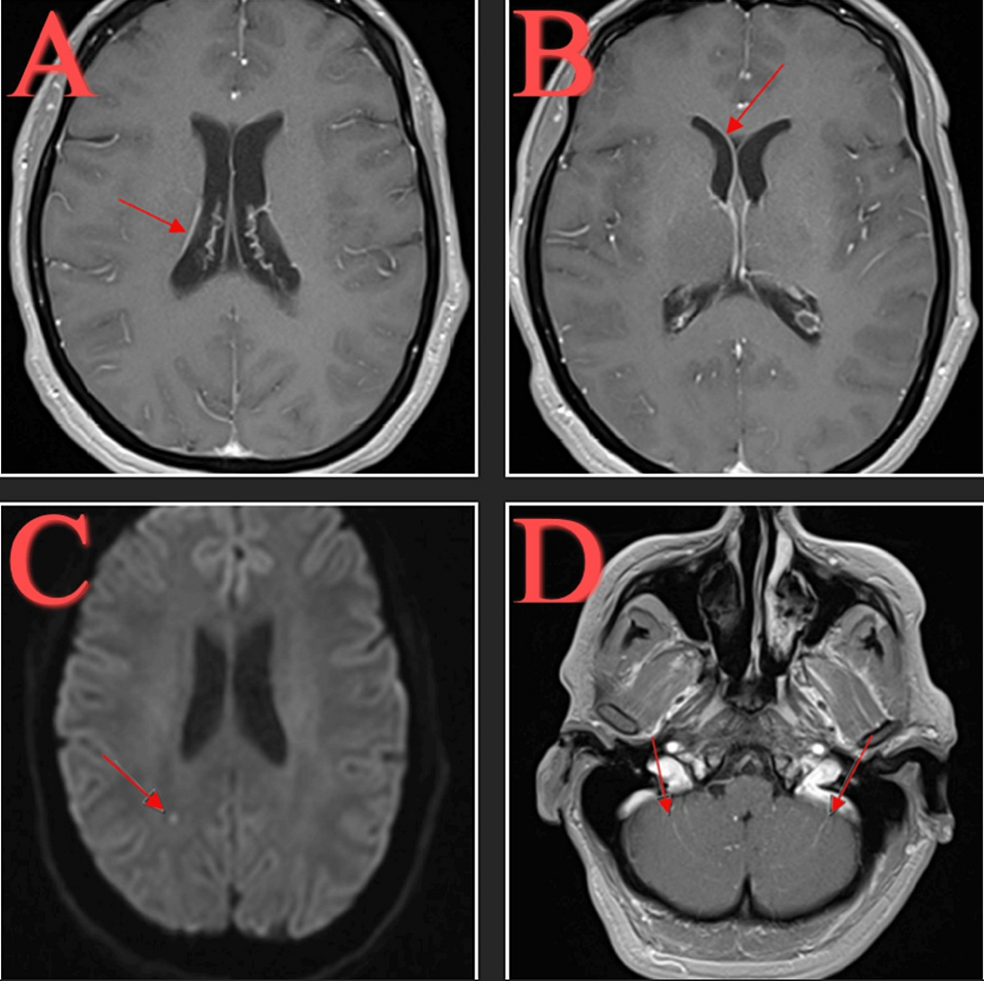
MRI brain with and without contrast. Mild enhancement of the ependymal lining of the ventricles (concerning ventriculitis) (A,B). Right posterior corona radiata acute lacunar infarct (C). Mild enhancement of the folia of the cerebellar hemispheres (D).
